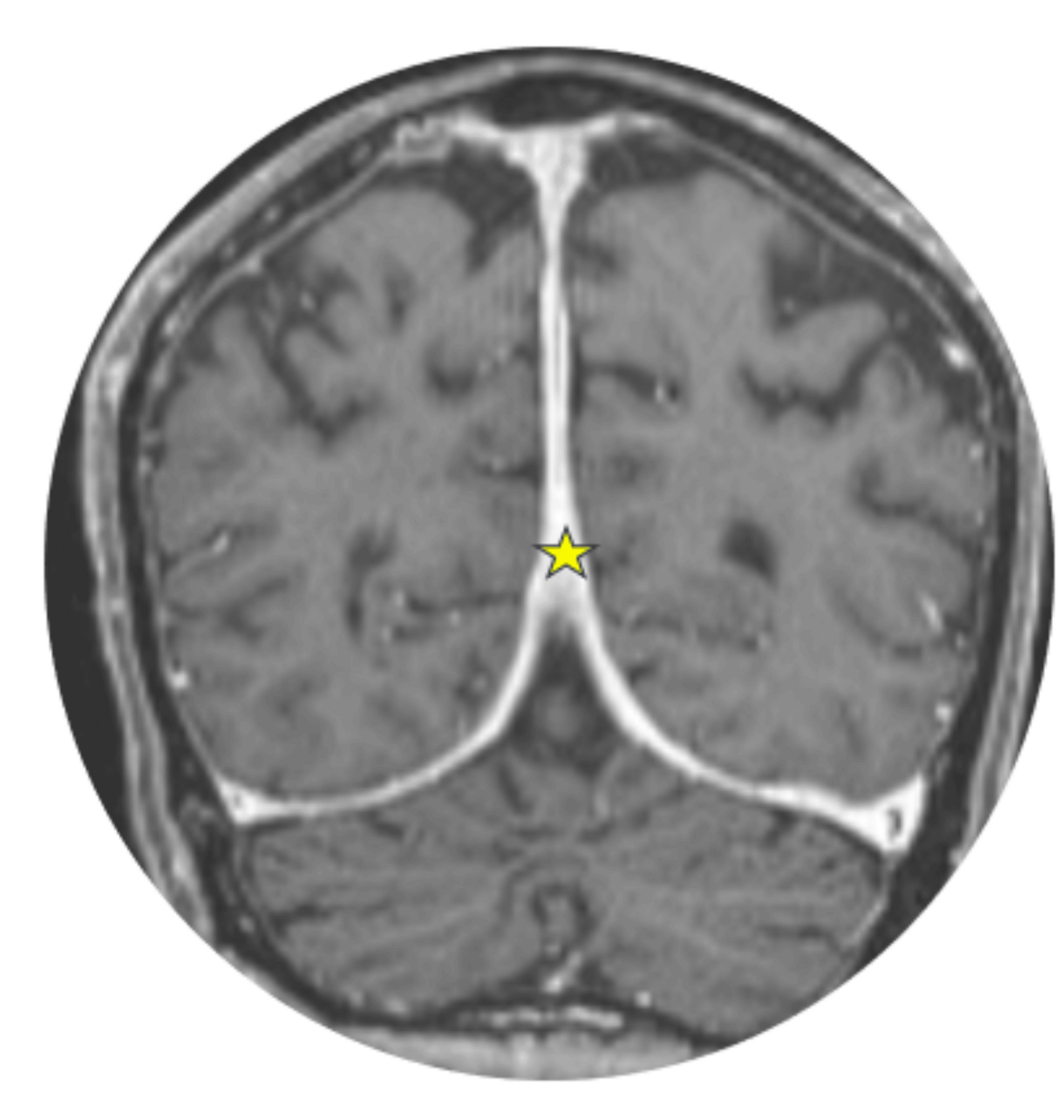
Coronal T1FS Gd MRI shows diffuse thick pachymeningeal enhancement (yellow star) MRI: magnetic resonance imaging; T1FS Gd: T1 fat-saturated post-gadolinium
